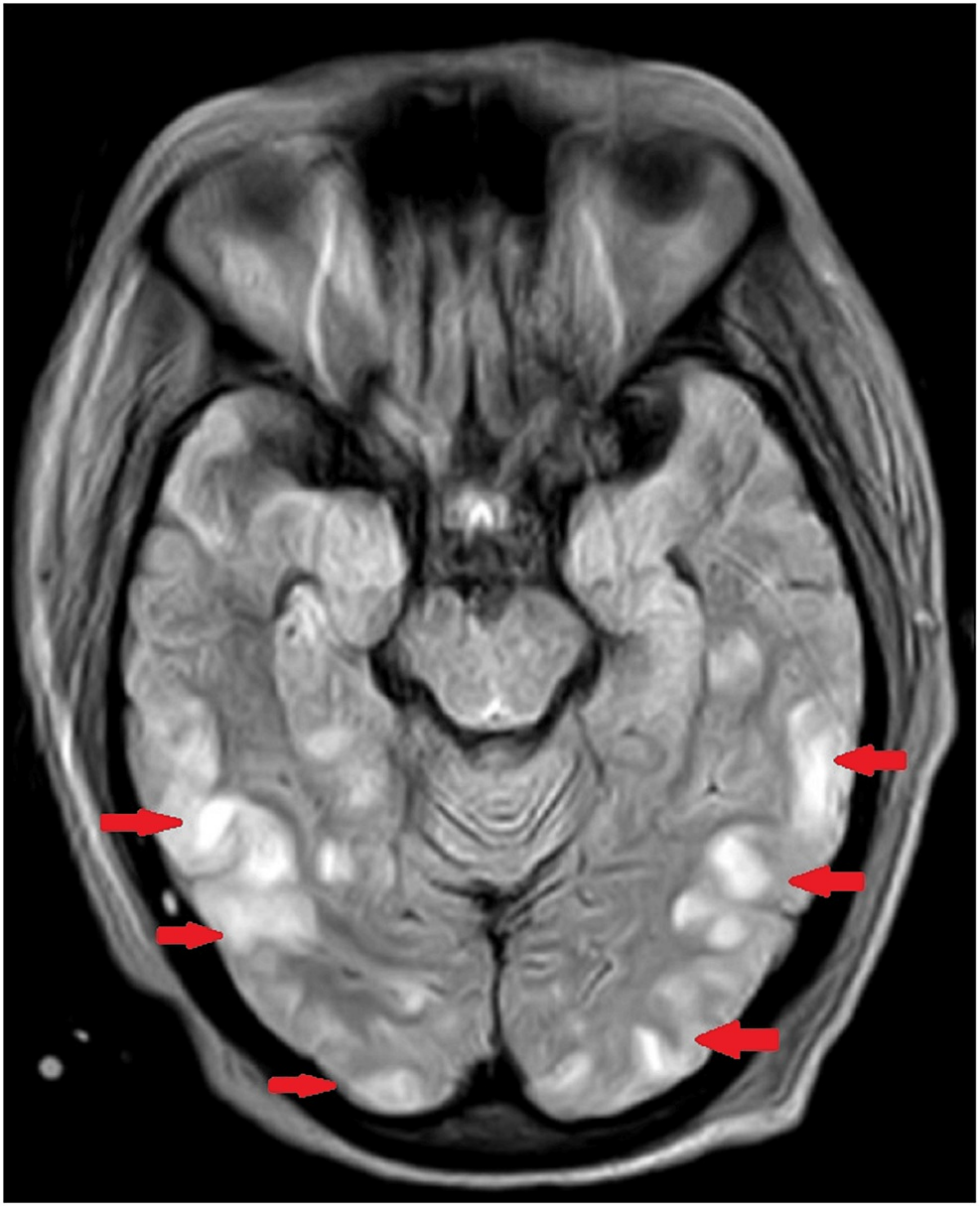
Bilateral parietal, temporal, and occipital white matter signal hyperintensities on MRI Brain T2/FLAIR sequence (red arrows) FLAIR: fluid-attenuated inversion recovery
